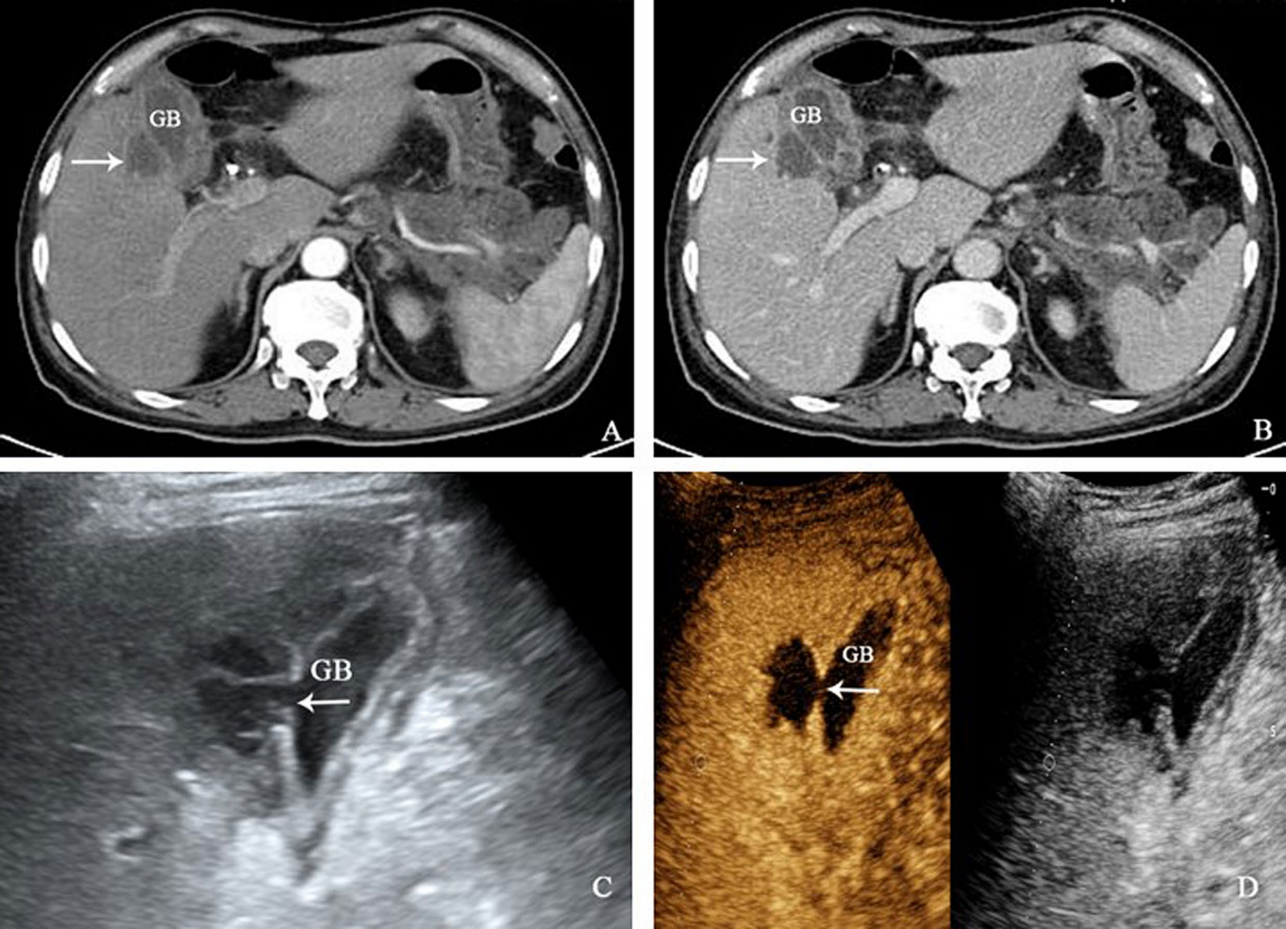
(A,B) The contrast-enhanced CT scan revealed thickening of the gallbladder wall, along with multiple cystic low-density shadows and enhanced edges within the wall. Furthermore, a 3.9 × 3.2 cm low-density mass with enhanced edges (white arrow) had formed in the right anterior lobe of the liver. (C) The right lateral wall of the gallbladder exhibited a discontinuity, with a local interruption in continuity of approximately 3.5 mm. Adjacent to the gallbladder wall, a mixed echo mass measuring about 4.2 × 3.4 cm was detected in the right anterior lobe of the liver. The margin between the liver mass and the gallbladder wall was indistinctly demarcated. (D) Following CEUS, the liver mass displayed uneven hyperenhancement during the arterial phase, along with obvious peripheral enhancement, hypoenhancement in the portal venous phase and delayed phase, and a non-enhanced area of 2.0 × 2.0 cm was observed within the liver mass.
